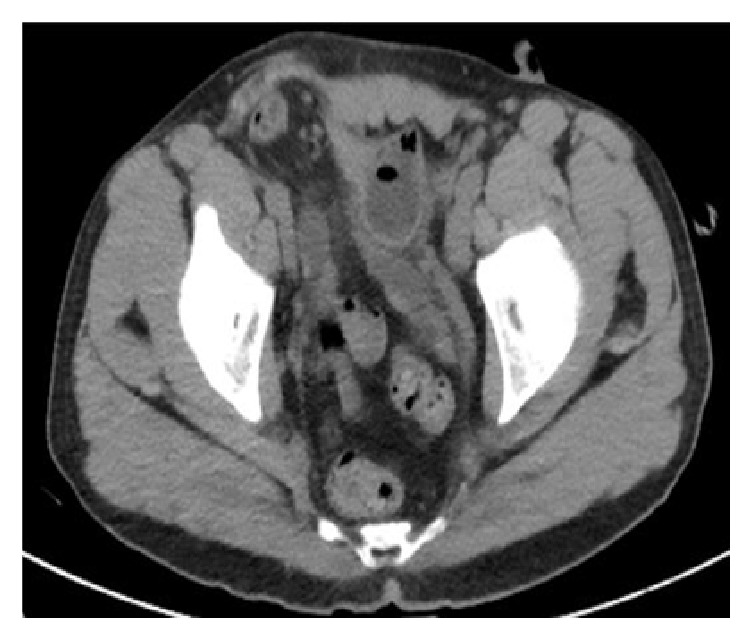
CT image demonstrates narrowing of bilateral dilated ureters entering the inguinal canal.
